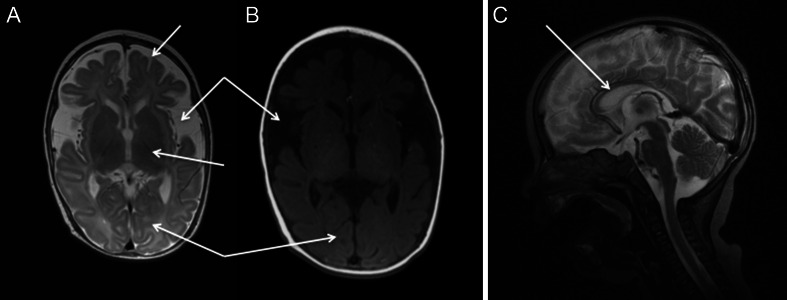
a-c. Axial and sagittal T_2_-weighted and T_1_-weighted cranial magnetic resonance imaging of a patient with type I ADSL deficiency: arrows indicate myelination of the posterior limbs of the internal capsules, enlarged subarachnoid space in the frontal regions, widened lateral ventricles (especially enlargement of the body and occipital horns), wide Sylvian fissures with hypoplasia operculum, thin corpus callosum and lack of myelination of the white matter volume
